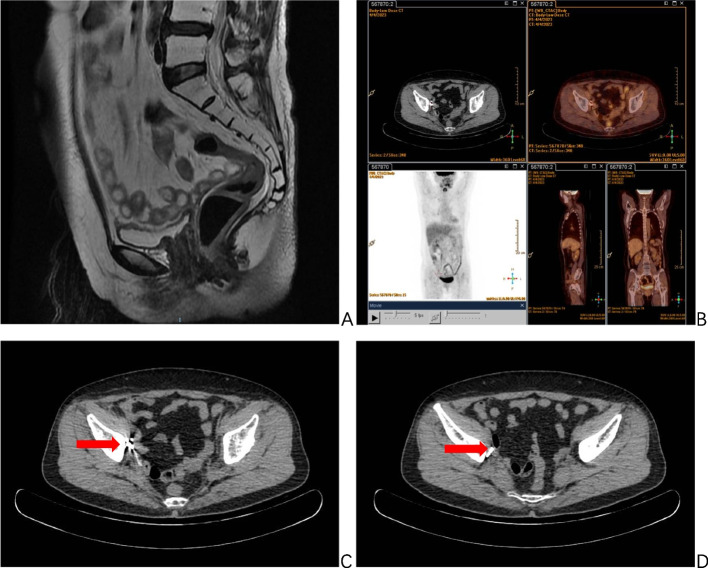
MRI and PET-CT examination after 125I seeds implantation. MRI examination about 6 months after 125I seeds implantation is shown in Figure A T2W sagittal position, the uterus, and bilateral adnexa were not shown, the uterus was altered postoperatively, and there were no definite signs of tumor recurrence in the operated area. PET-CT examination 13 months after 125I seeds implantation is shown in Figure B multiple punctate dense shadows on the right wall of the pelvis, and no swollen lymph nodes were seen in the pelvis and bilateral inguinal area, and bilateral inguinal area showed no enlarged lymph nodes. Figures C and D multiple punctate dense shadows in the right wall of the pelvis (indicated by arrows), which are seeds implantation changes
